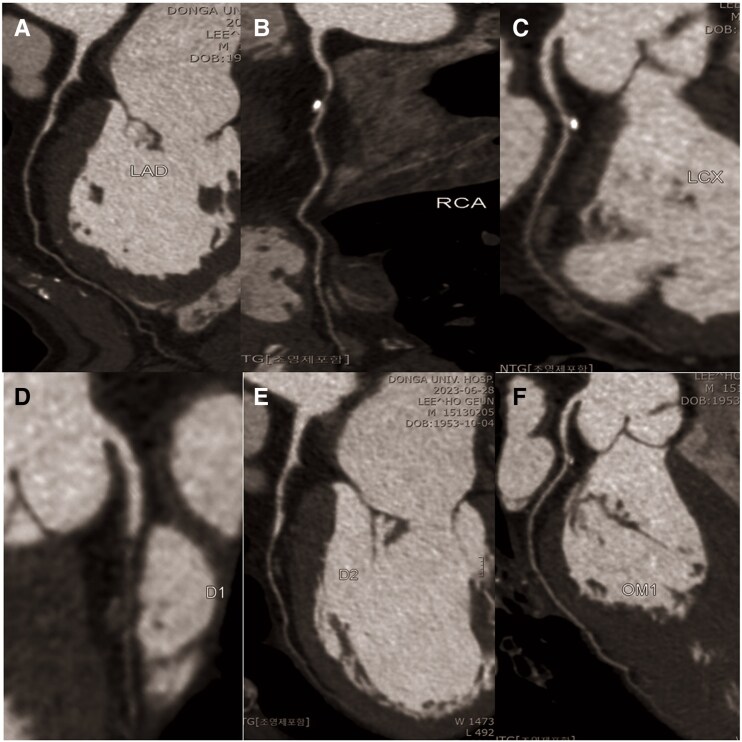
Case 2: curved multiplanar reformation imaging from multi-detector computed tomographic coronary angiography. (*A*) Left anterior descending artery. (*B*) Right coronary artery. (*C*) Left circumflex artery. (*D*) First diagonal branch. (*E*) Second diagonal branch. (*F*) Obtuse marginal branch.
